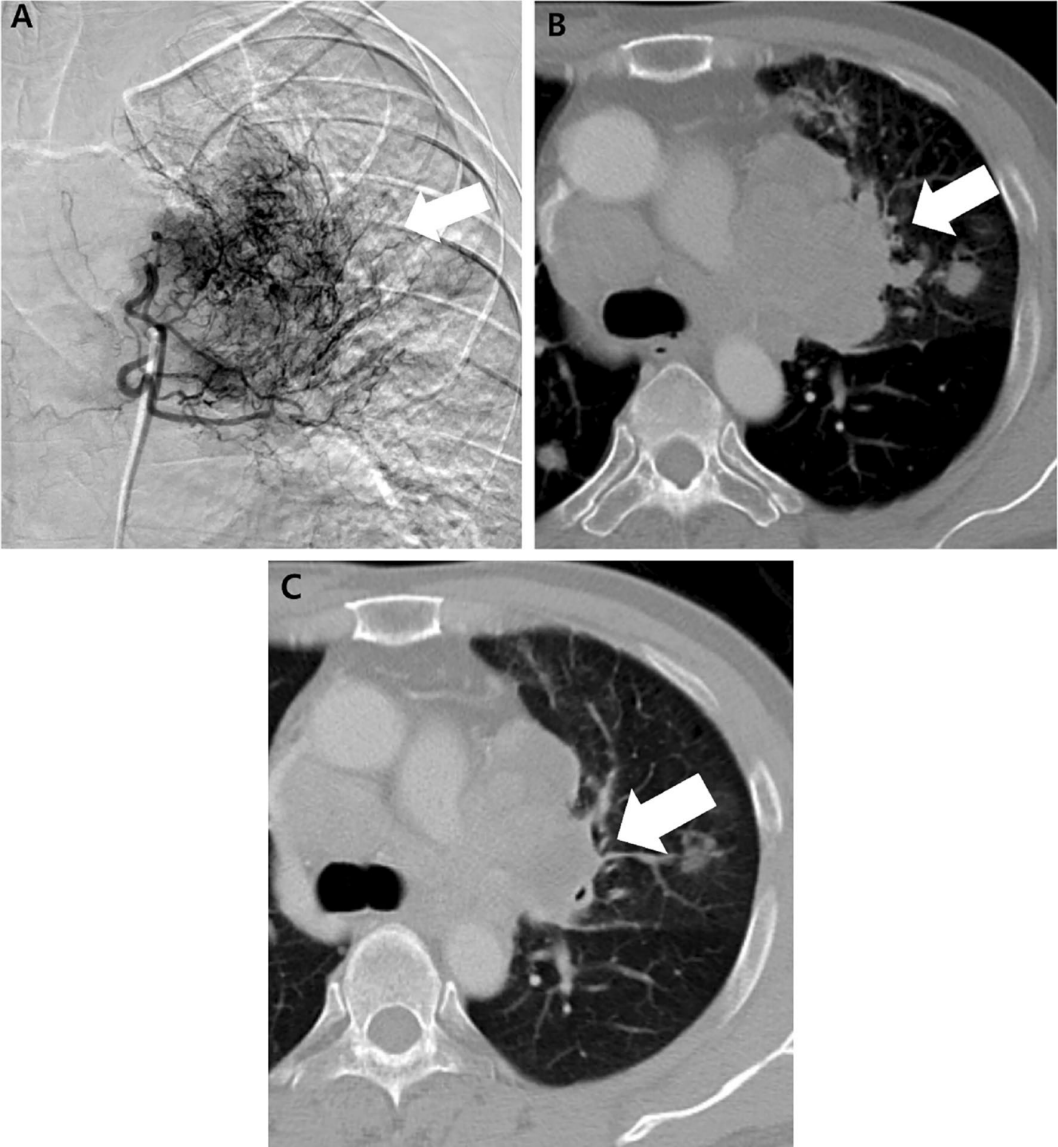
A 63-year-old man with a pulmonary metastasis from HCC who presented with a massive amount of hemoptysis. (A) Selective left bronchial arteriography using a 5-F catheter shows a tortuous hypertrophic left bronchial artery and a large hypervascular tumor blush (white arrow). Embolization of the left bronchial artery was successfully performed using gelfoam, and this patient showed complete resolution of hemoptysis after bronchial artery embolization. (B) Axial CT image before embolization shows a huge metastatic mass (white arrow) in the left upper lobe. (C) Axial CT image 3 months after embolization of the bronchial artery shows a marked decrease in the size of the metastatic mass (white arrow) in the left upper lobe.
